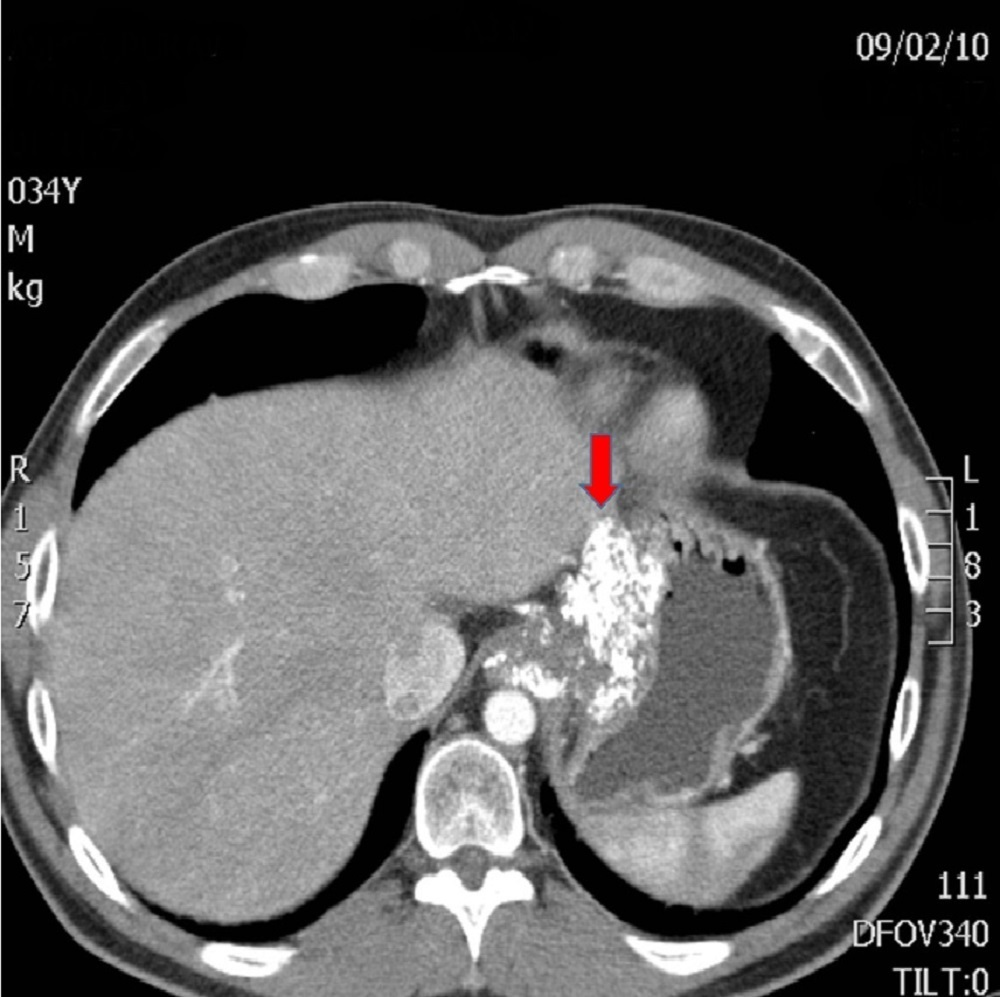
CT and MRI Preoperative Images Axial CT - loss of fat plane between stomach and left lobe of liver indicating possible infiltration by tumor (arrow)
